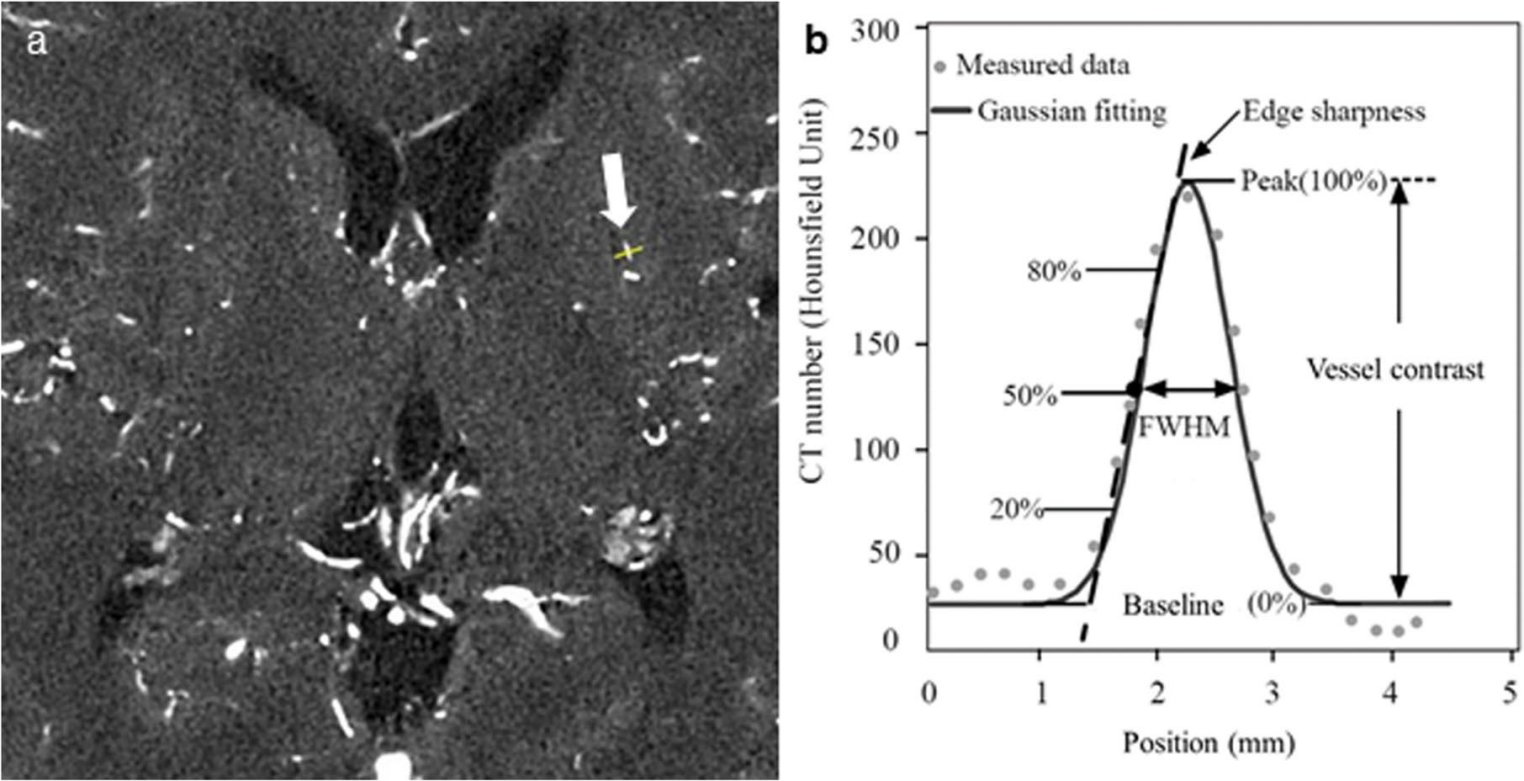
Measurements of edge sharpness, peak CT number, vessel contrast, and FWHM. a A linear region of interest is positioned perpendicular to the target vessel on the source image. The arrow indicates a moyamoya perforator vessel. The locations of regions of interest varied among the patients. b Measurements were taken on the Gaussian-fitted line profile. Edge sharpness was defined as the slope of a line passing through 20% and 80% of the peak CT number. Vessel contrast was defined as the difference between the peak (100%) and baseline (0%) CT numbers. *FWHM* full width at half maximum
